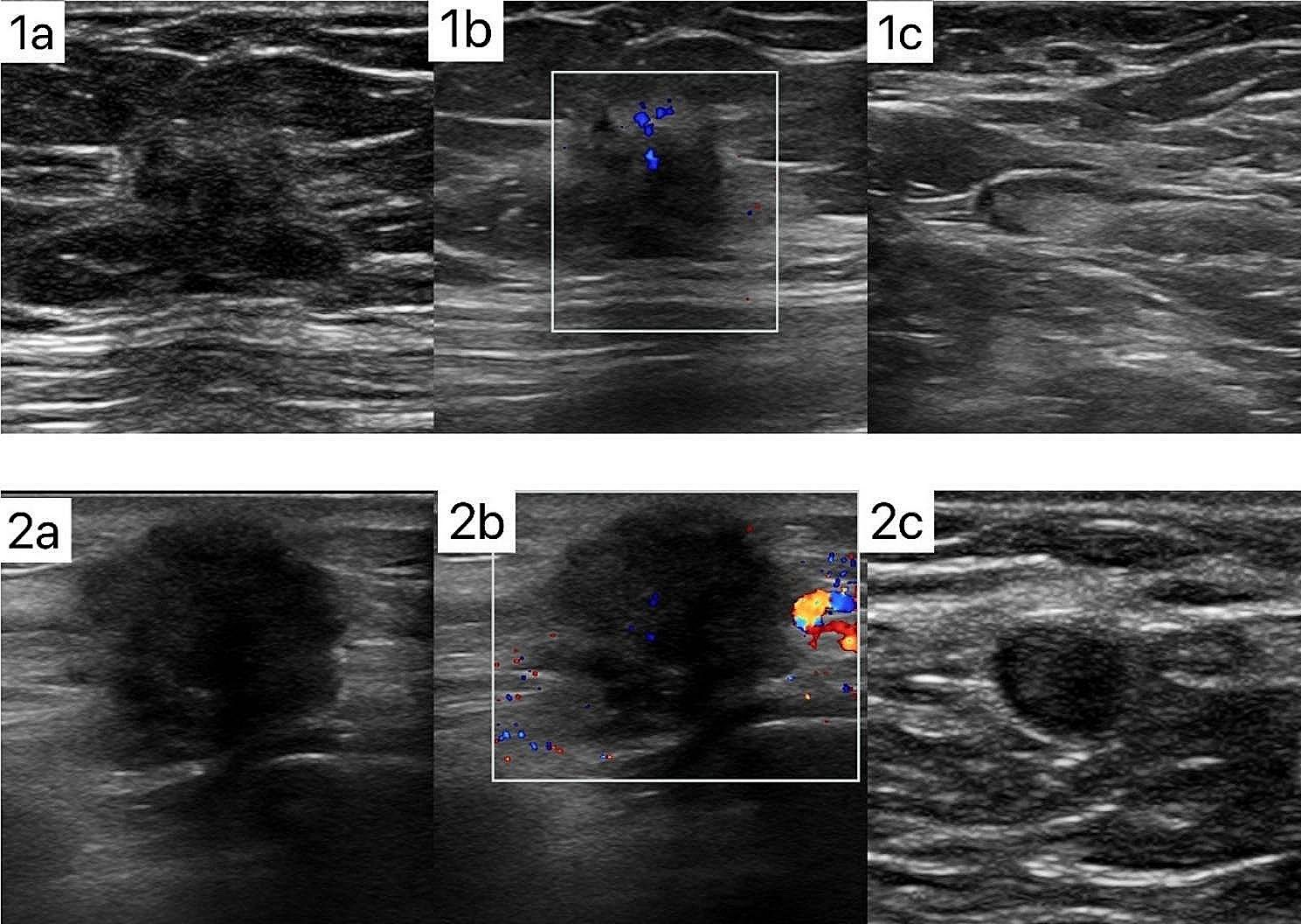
1a. US image of 31-year-old woman in stage I (MD = 1.2 cm) breast cancer with limited lymph node burden. US detected a hypoechoic lesion on outer quadrant in the right breast with irregular shape, indistinct margin, posterior no feature and hyperechoic halo. 1b. CDFI: A small amount of punctate blood flow signal is visible. 1c. Axillary ultrasound shows normal lymph nodes. 2a. US image of 43-year-old woman in stage II (MD = 2.8 cm) breast cancer with high lymph node burden. US detected a hypoechoic lesion on outer quadrant in the left breast with irregular shape, spiculated margin, posterior feature shadowing and hyperechoic halo. 2b. CDFI: penetrating branch blood flow signal is visible. 2c. Axillary ultrasound shows suspicious lymph nodes
